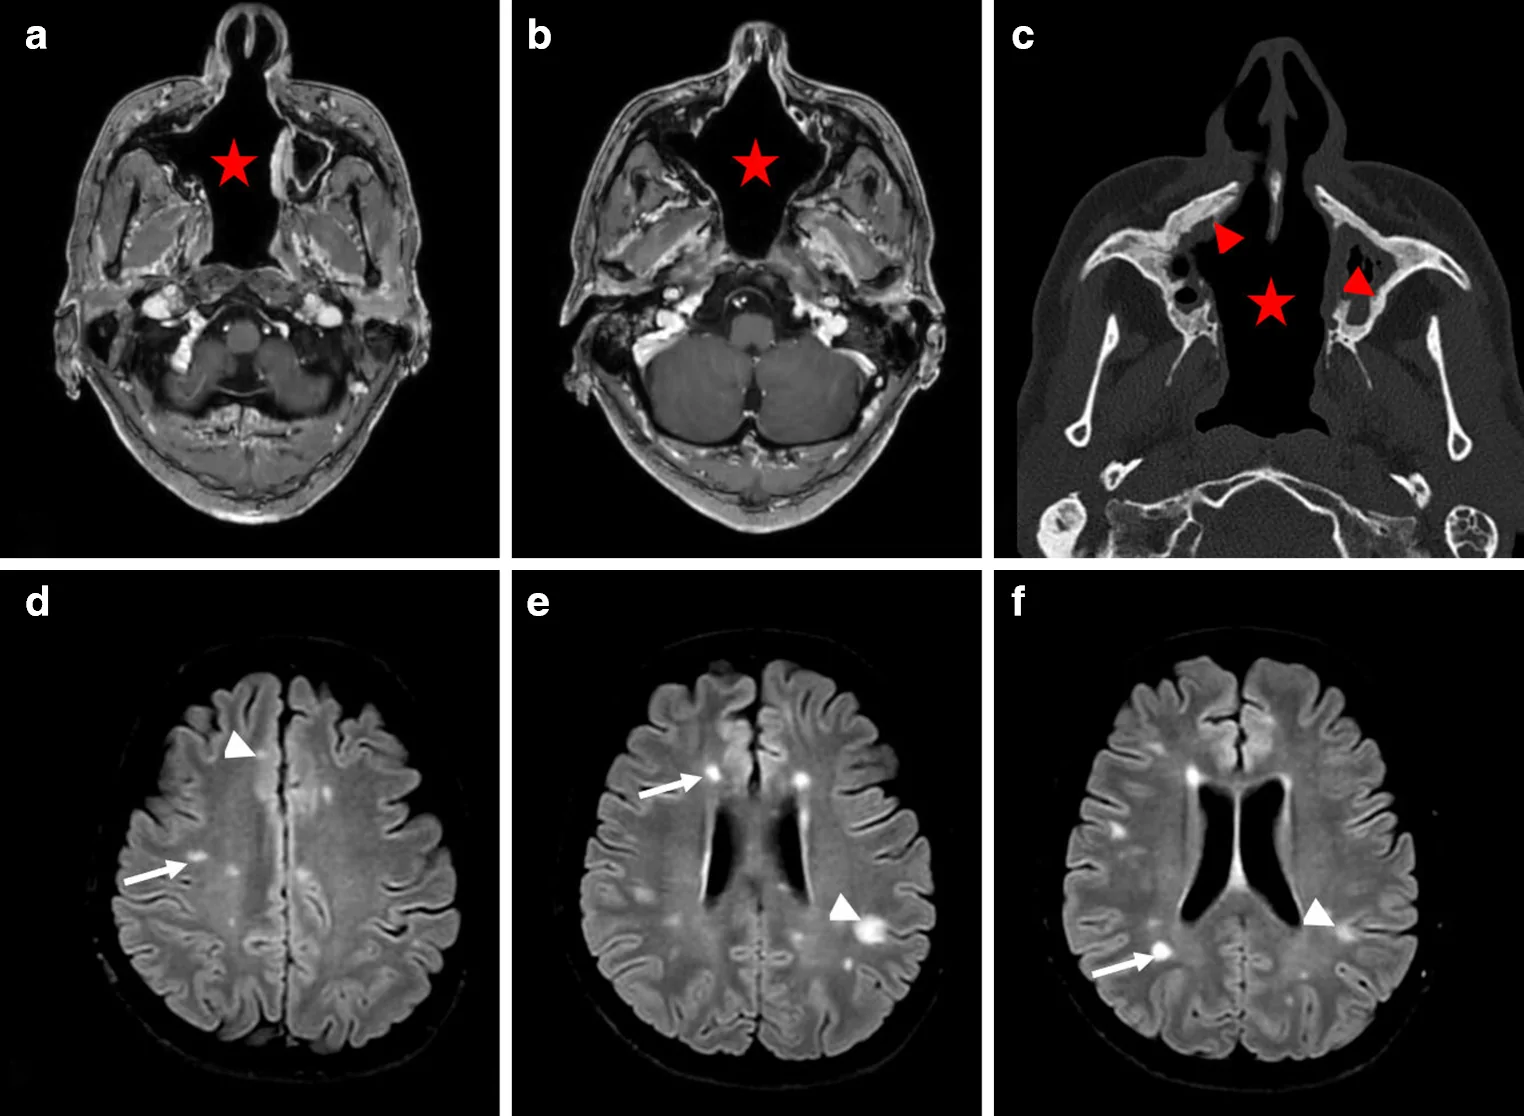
Granulomatosis with polyangiitis (GPA; Wegener’s granulomatosis). a–c Extended destruction of the paranasal sinuses and the nasal septum (a, b post contrast T1 WI; c CT; asterisk) with increased sclerosis of adjacent bony structures (c arrowhead); d–f fluid attenuated inversion recovery (FLAIR) images showing numerous subcortical (d–f arrow) and also juxta-/cortical lesions (d–f arrowhead)
